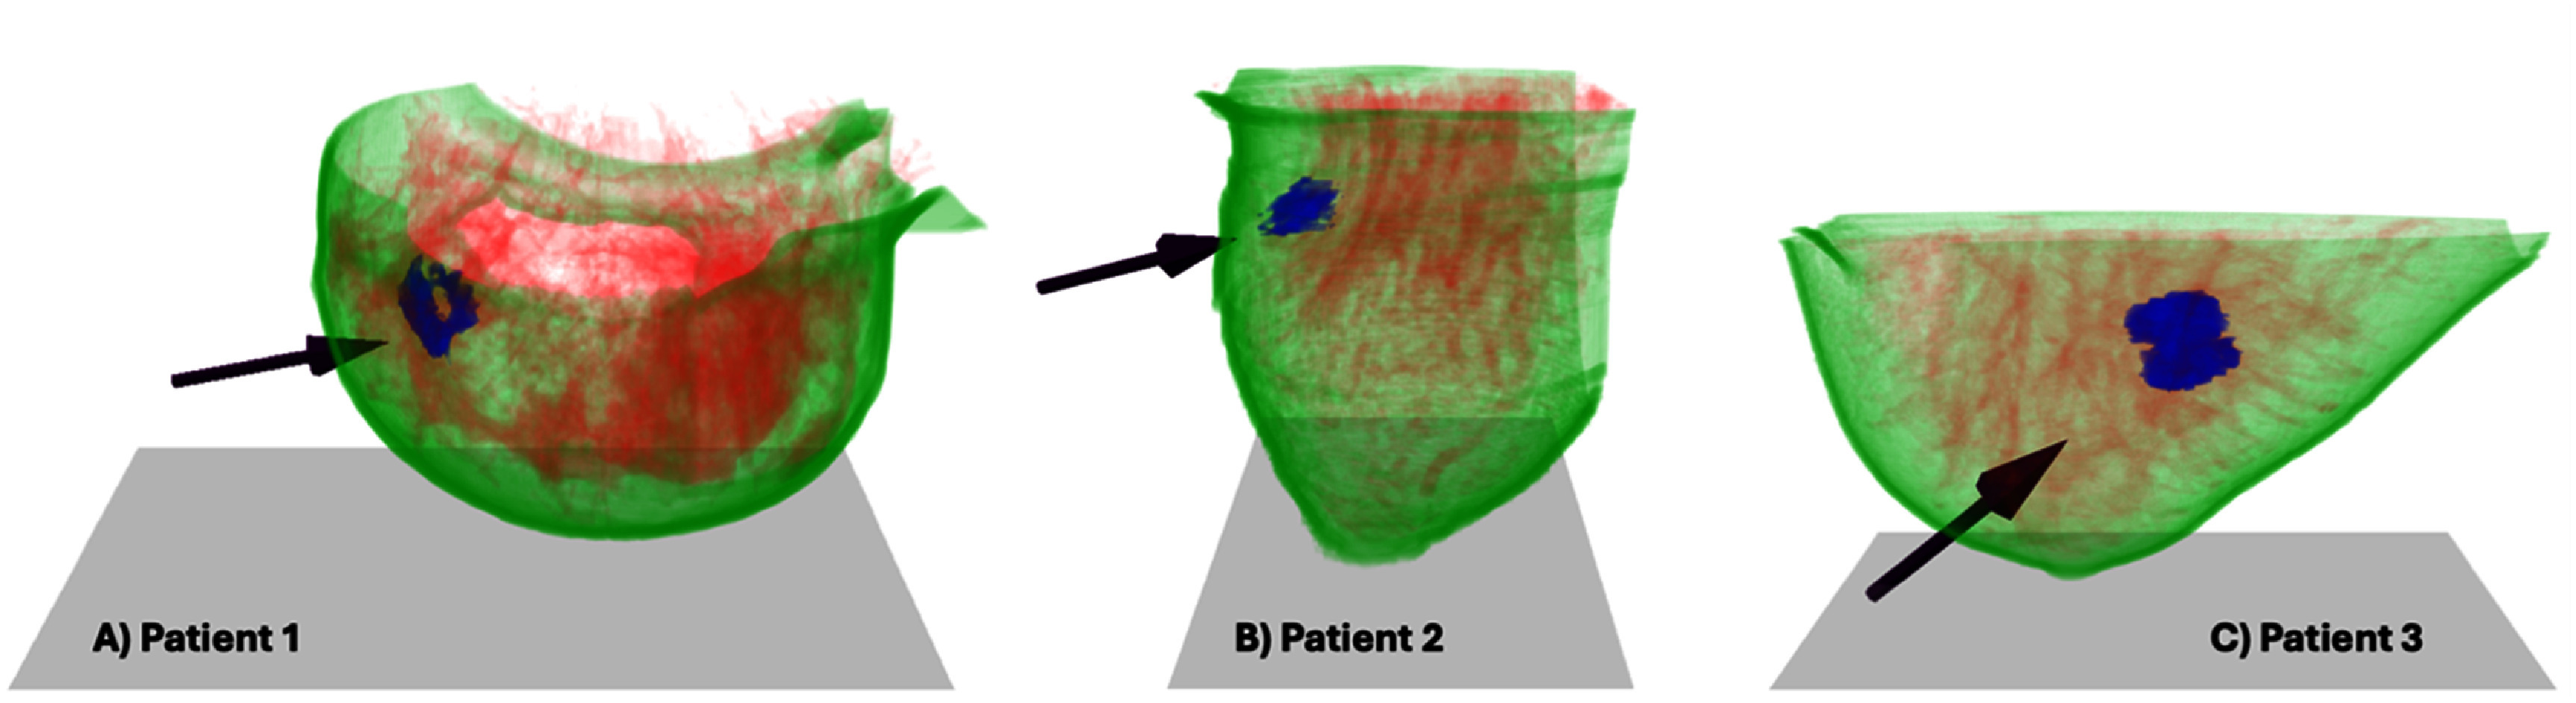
3D-rendering of tumor (blue), fibroglandular tissue (red), and skin (green) in A) Patient 1, B) Patient 2, and C) Patient 3. Arrows indicate the ultrasound propagation direction.
